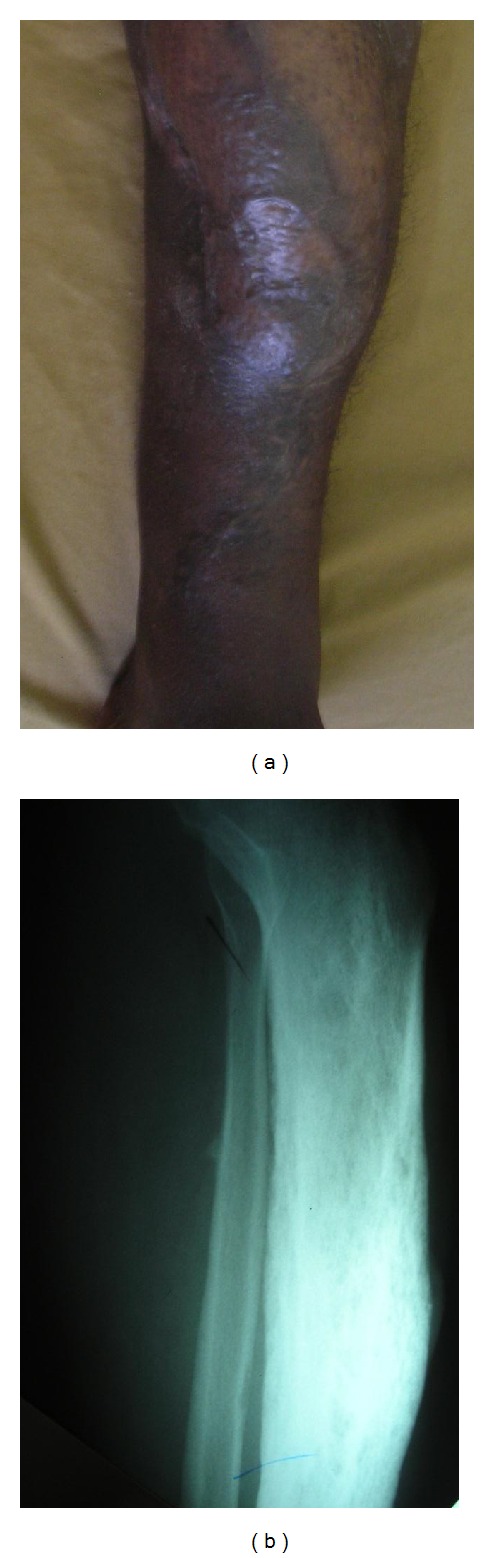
(a) shows the good take of the skin graft and gastrocsoleus flaps; the sinuses have not recurred. (b) Lateral X-ray of the left tibia-fibula showing the resolution of the lytic defects in the tibia.
